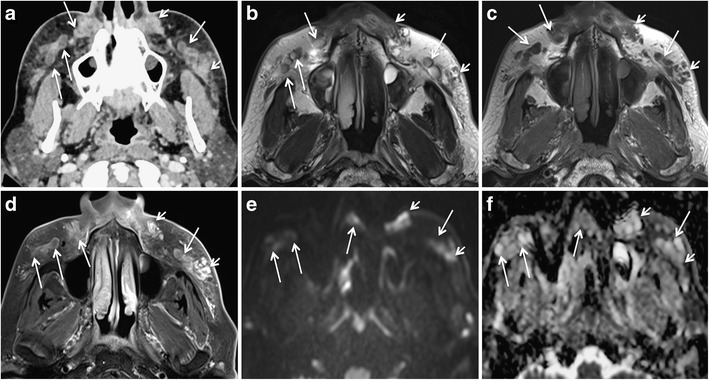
A 65-year-old woman developed pain, erythema, and bilateral cheek swelling after 6 months of HA facial filler injections. a Contrast-enhanced CT shows bilateral “grape-like” hypodense, rim-enhancing areas (*long arrows*) and solid appearing enhancing nodules (*short arrows*) in the nasolabial fat, medial and middle superficial cheek fat compartments. T2 W (b), T1 W (c), and fat-saturated gadolinium enhanced T1 W (d) images reveal that the rim-enhancing areas already seen on CT have a high protein content (hypo-isointense on T2 and T1). On b1000 (e) and ADC map (f), these rim-enhancing lesions show variable diffusivity (long arrows). ADC values were between 1.2 and 2 × 10^−3^ mm^2^/s. The solid lesions (*short arrows*) show strong enhancement on MRI and no restricted diffusivity. Surgery confirmed bilateral infected fluid collections and isolated FBG
